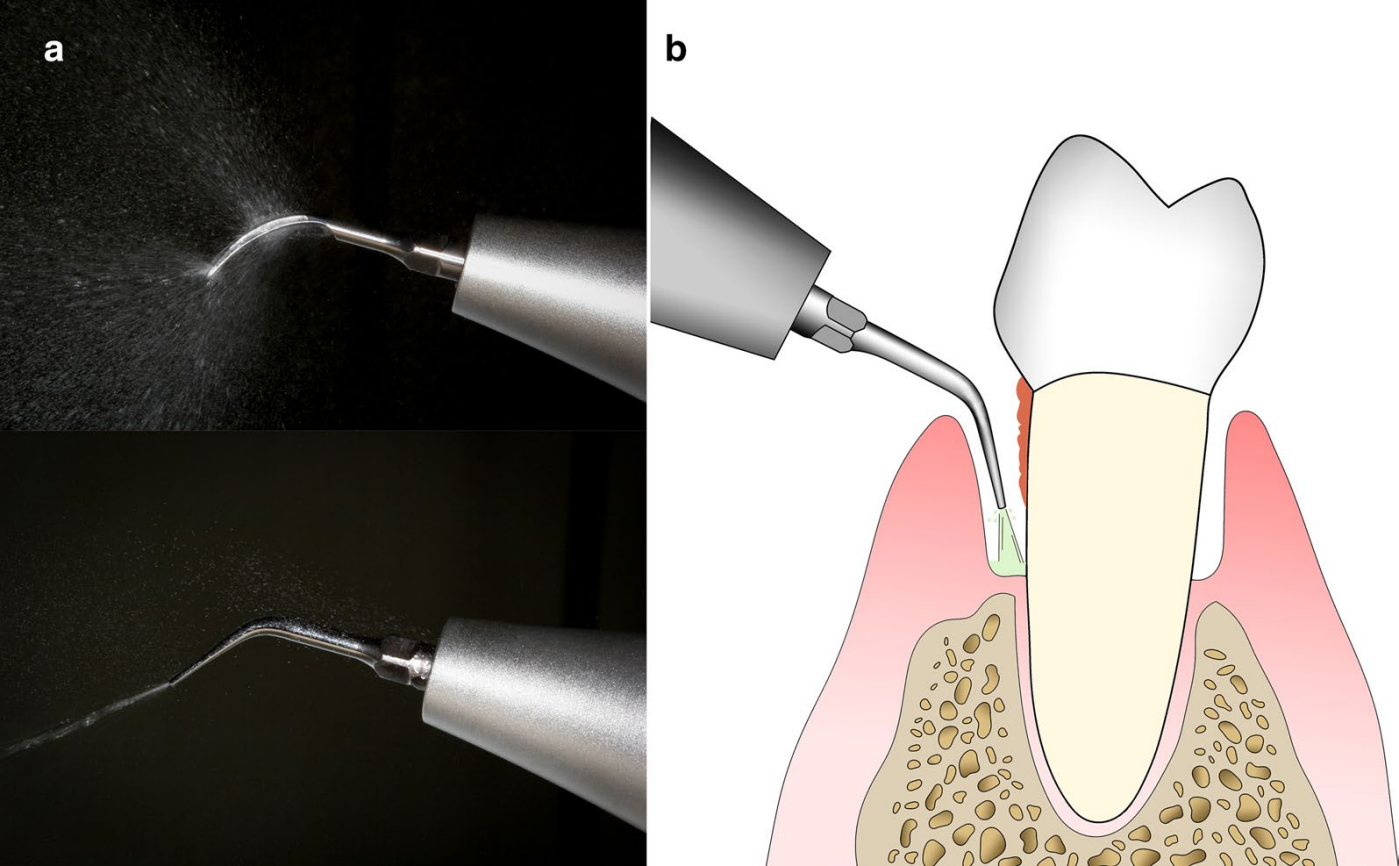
The ultrasonic scaler tips used in this study. a The conventional P3 (up) and new-type scaler tip (down), b terminal outlet of the new-type scaler tip allows coolants to be ejected into deep pockets
